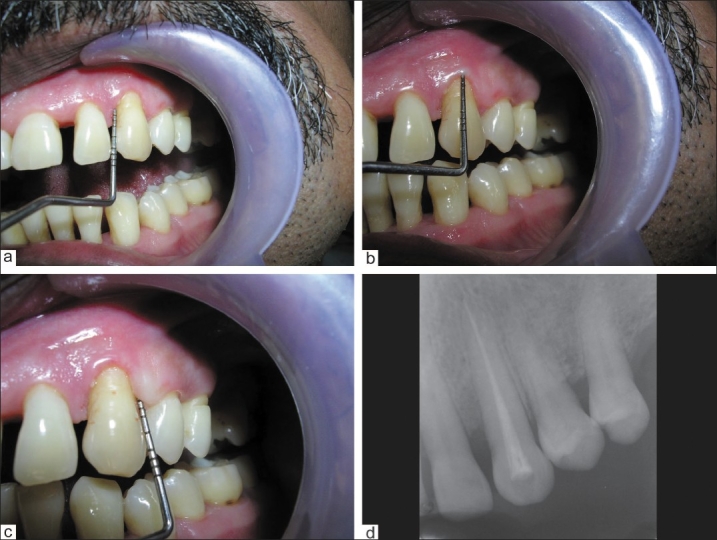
a) The periodontal pockets had reduced from 8 mm to 0.5 mm mesially; b) The periodontal pockets had reduced from 6 mm to 1 mm labially; c) The periodontal pockets had reduced from 7.5 mm to 1 mm distally; d) Radiographically, a significant bony fill was evident after nine months
